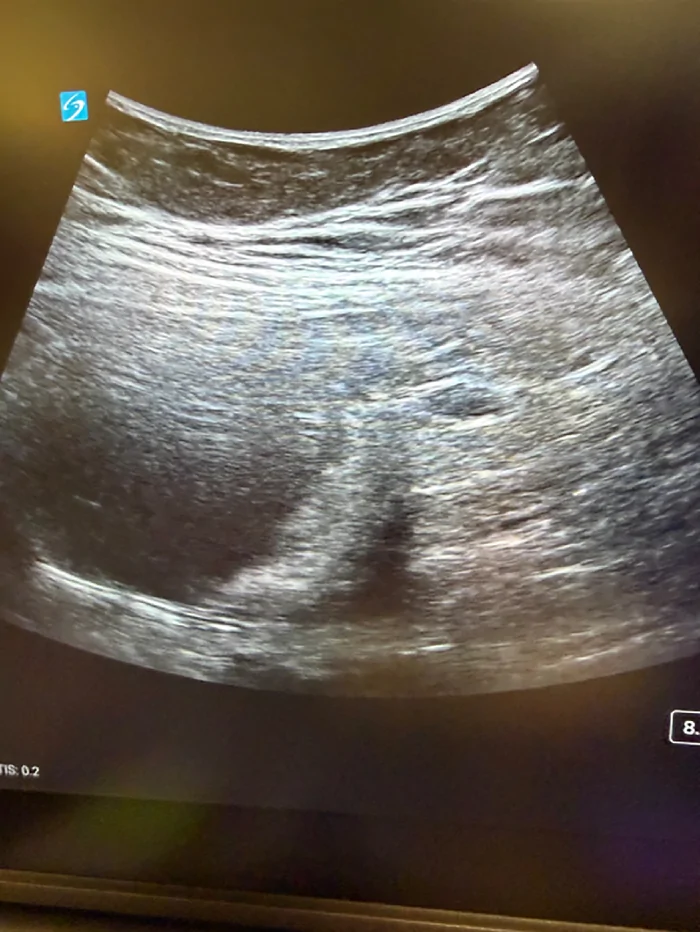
Gastric ultrasound of the stomach with the patient in the supine position. Both hypoechoic and hyperechoic material were identified, a grade 2 finding.
